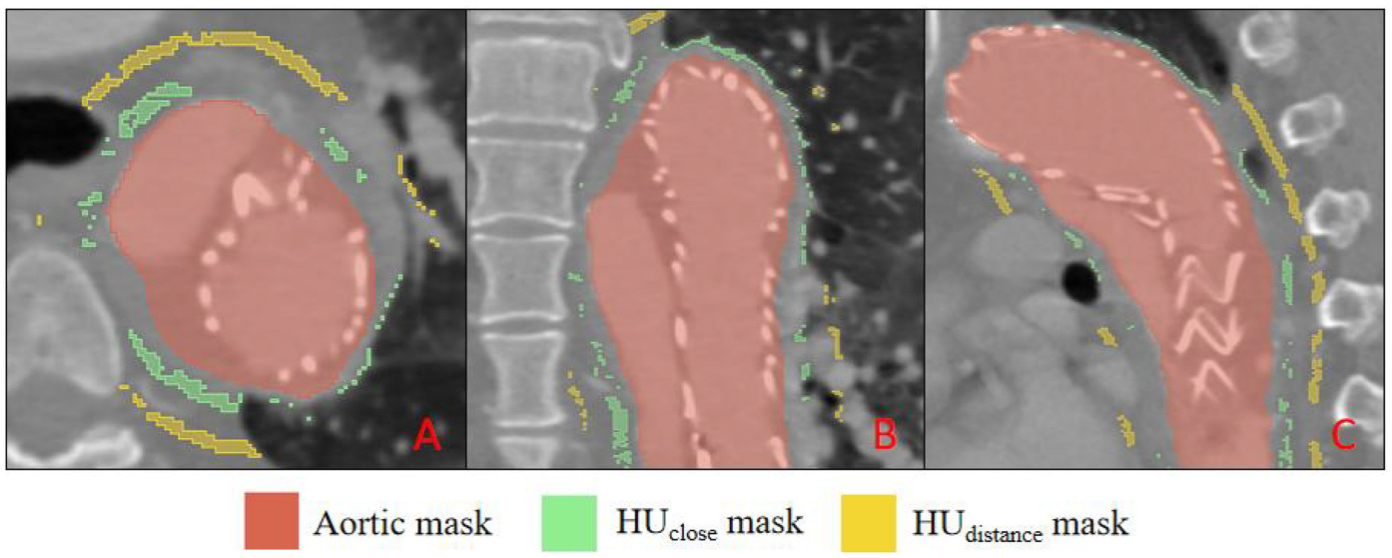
Multiplanar CT segmentation of perivascular adipose tissue (PVAT). (A) shows an axial (transverse) slice, (B) a coronal slice, and C a sagittal slice. The aortic lumen is delineated in red (aortic mask); PVAT immediately adjacent to the aortic wall is highlighted in green (HU close mask), while PVAT located farther from the wall is indicated in yellow (HU distance mask).
